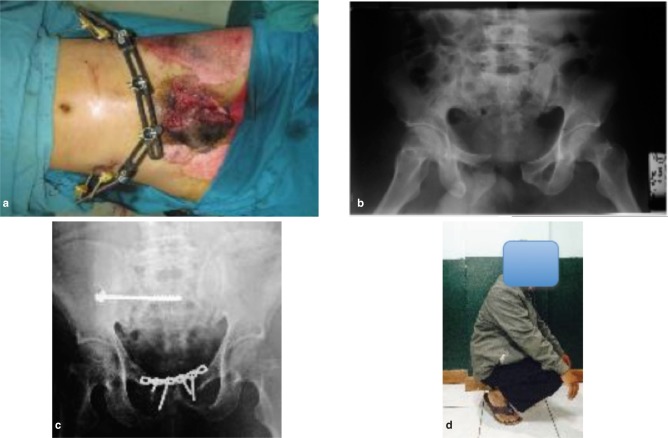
Open Pelvic fracture MTB 1. (a) clinical picture (b) pre operative radiograph (c) post operative radiograph (d) 1 year after operation with excellent majeed pelvic score.
